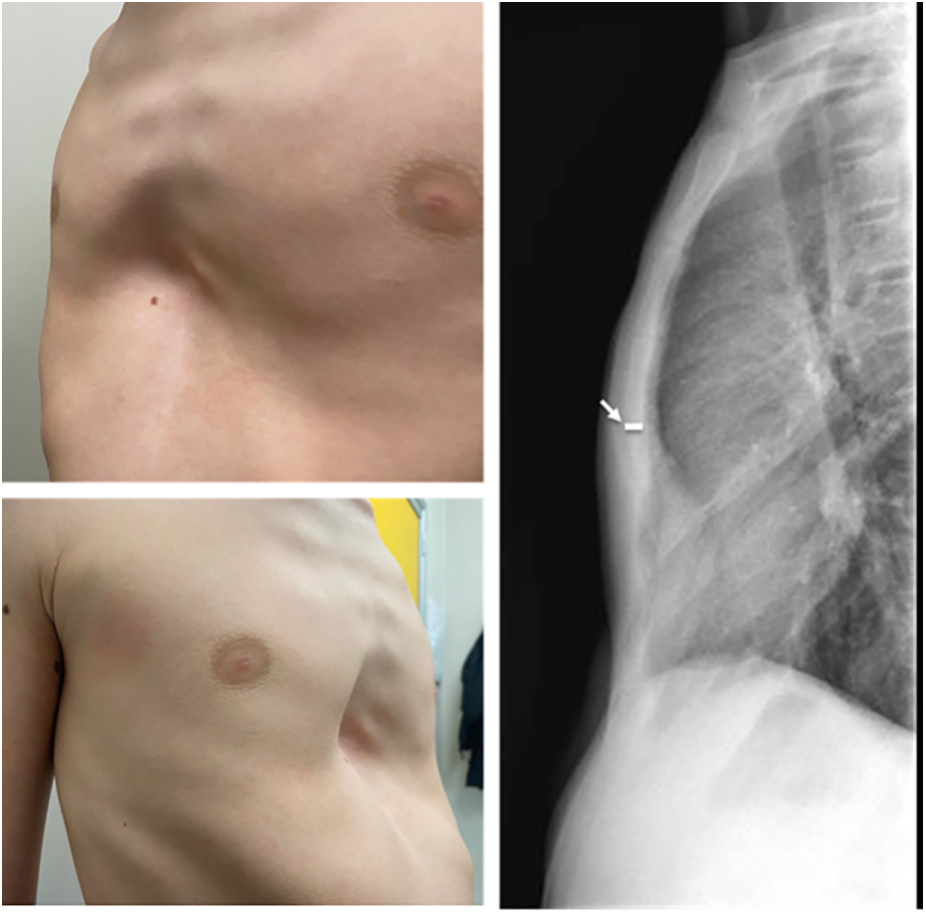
X-ray chest to evaluate the thickness of the sternum.
